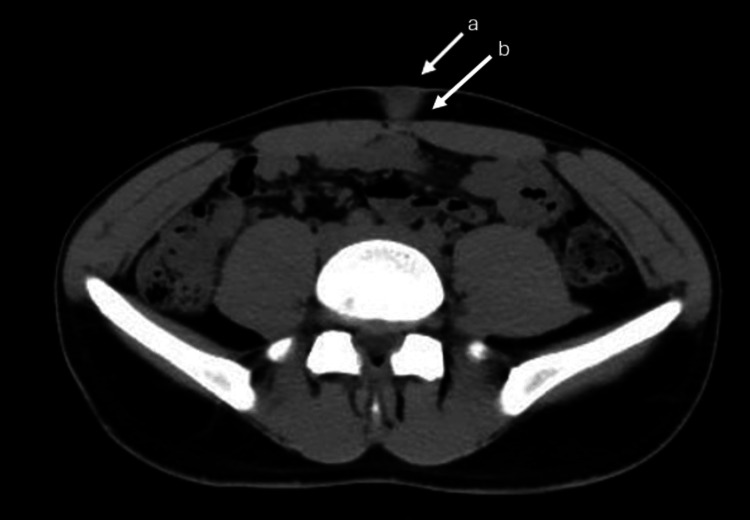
(a) Homogenous granuloma-like umbilical soft tissue mass seen in abdominal computed tomography; (b) thin rim separating the umbilical soft tissue mass from the underlying abdominal muscles.
